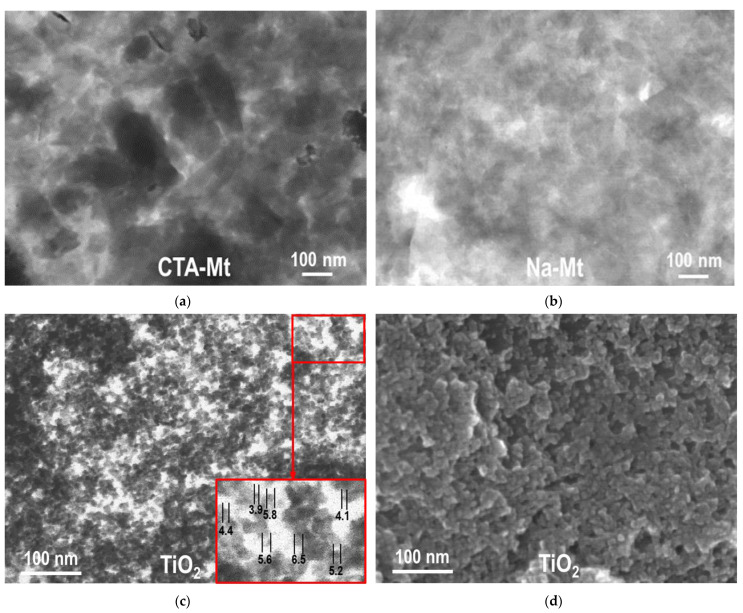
(a) TEM image of CTA-Mt; (b) TEM image of Na-Mt; (c) TEM image of titania obtained by inverse microemulsion method; (d) SEM image of titania obtained by inverse microemulsion method.
